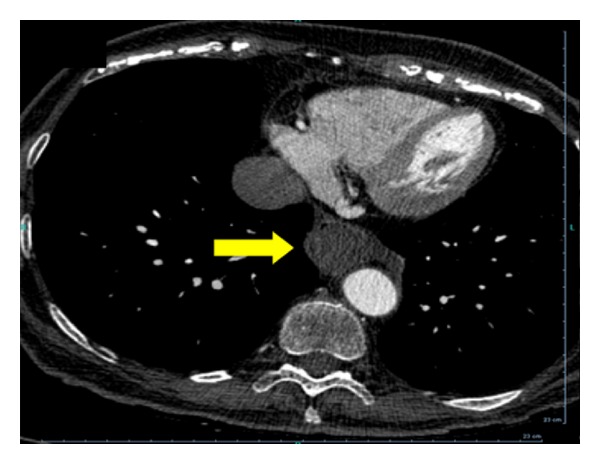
CT of distal esophagus revealing near-complete occlusion of the lumen (arrow).
